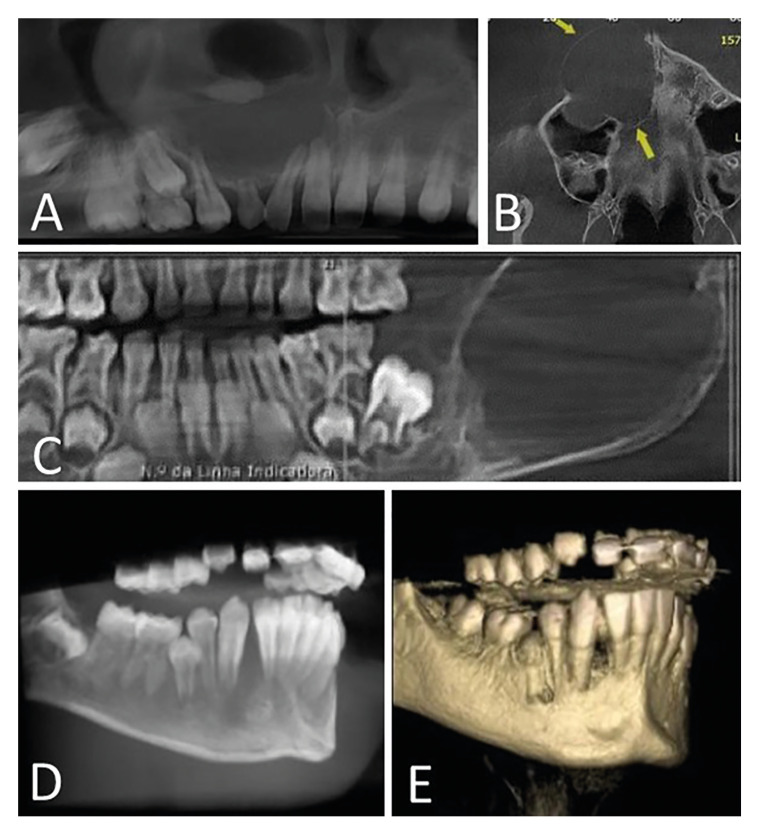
Radiographic aspect. A) CT image of a 14-year-old female patient revealed an expansive lesion with well-delineated borders in the maxilla causing displacement of the tooth and root resorption. B) An axial CT image of the same case showing a lesion with thinning of the cortical bone due to bony expansion (yellow arrows). C) CT image of a 5-year-old female patient presenting with an expansive lesion in the posterior region of the mandible causing thinning of the cortical bone. D) CT image of an 11-year-old male patient with a destructive lesion in the anterior mandible, causing bone resorption near the mental foramen associated with associated paresthesia. E) 3D reconstruction CT scan of the same patient.
